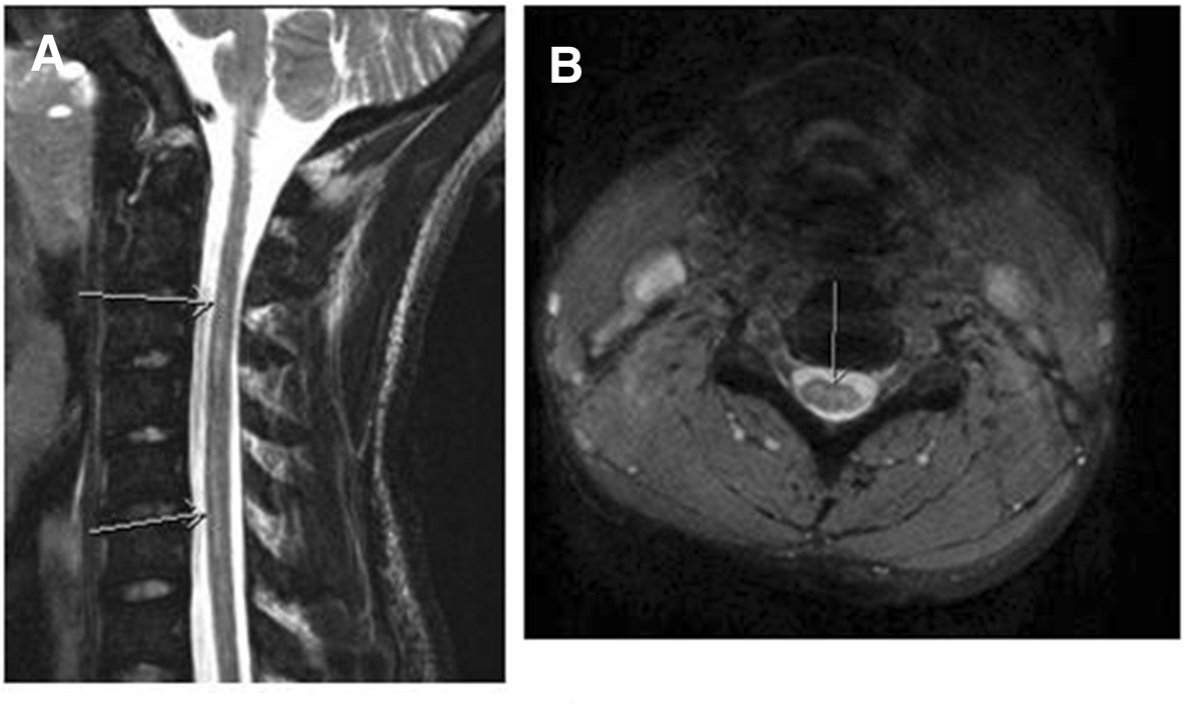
A,B Follow-up magnetic resonance imaging study demonstrating no spinal cord atrophy with residual signal from myelomalacia, months after infarct. Arrows point to the decrease in edema.
